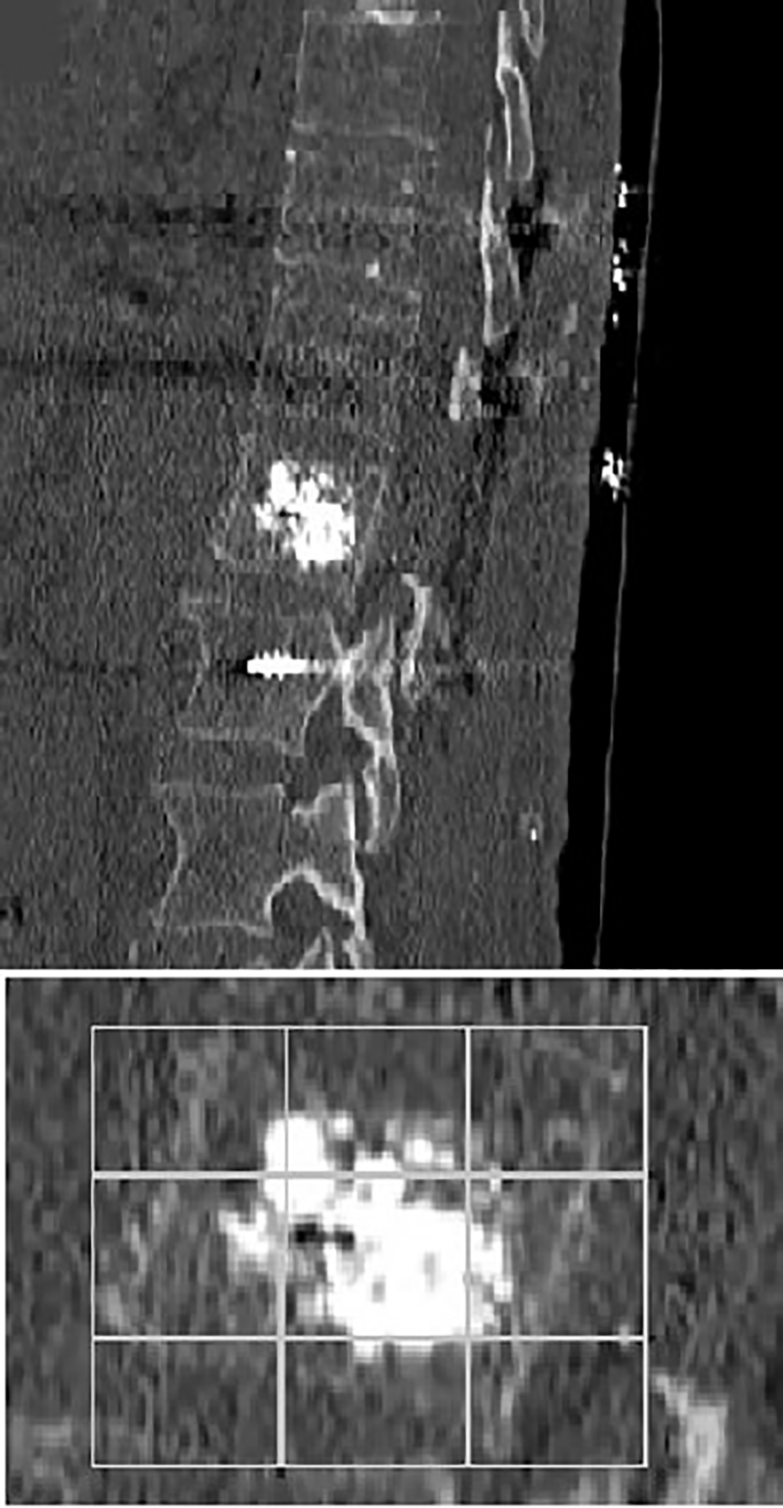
Sagittal early post-operative CT scan showing an L1 average cement filling based on Saliou filling score 11/18 (6 + 5) and a T11-T12-L2 vertebral fixation.
